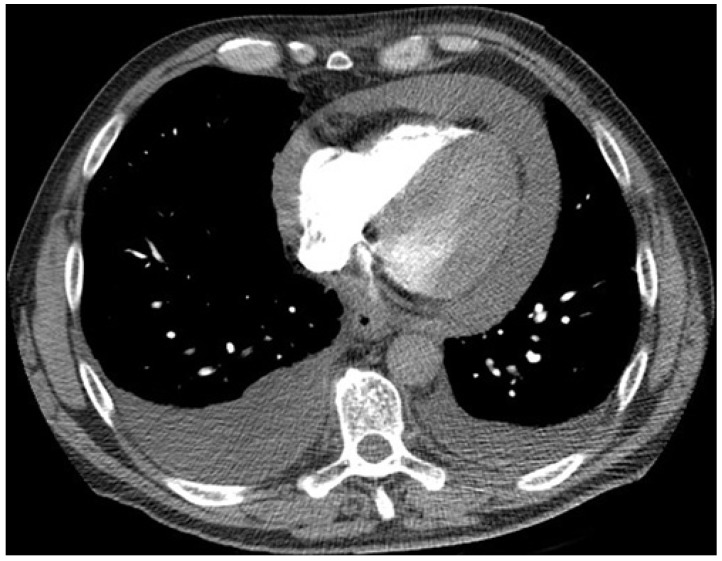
Computed tomography (CT) chest axial view. Large pericardial effusion and moderate bilateral pleural effusions.
